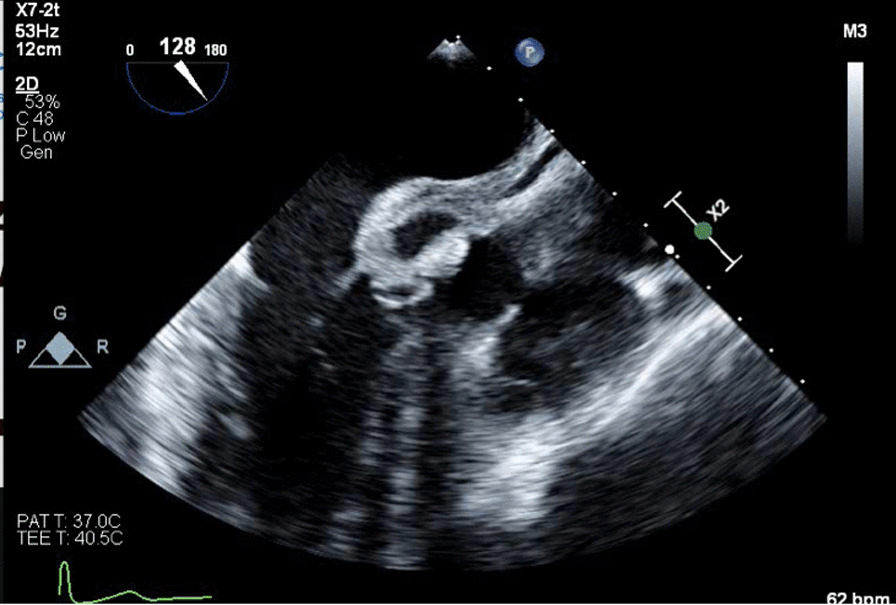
Transesophageal echocardiogram demonstrating aortic root abscess
